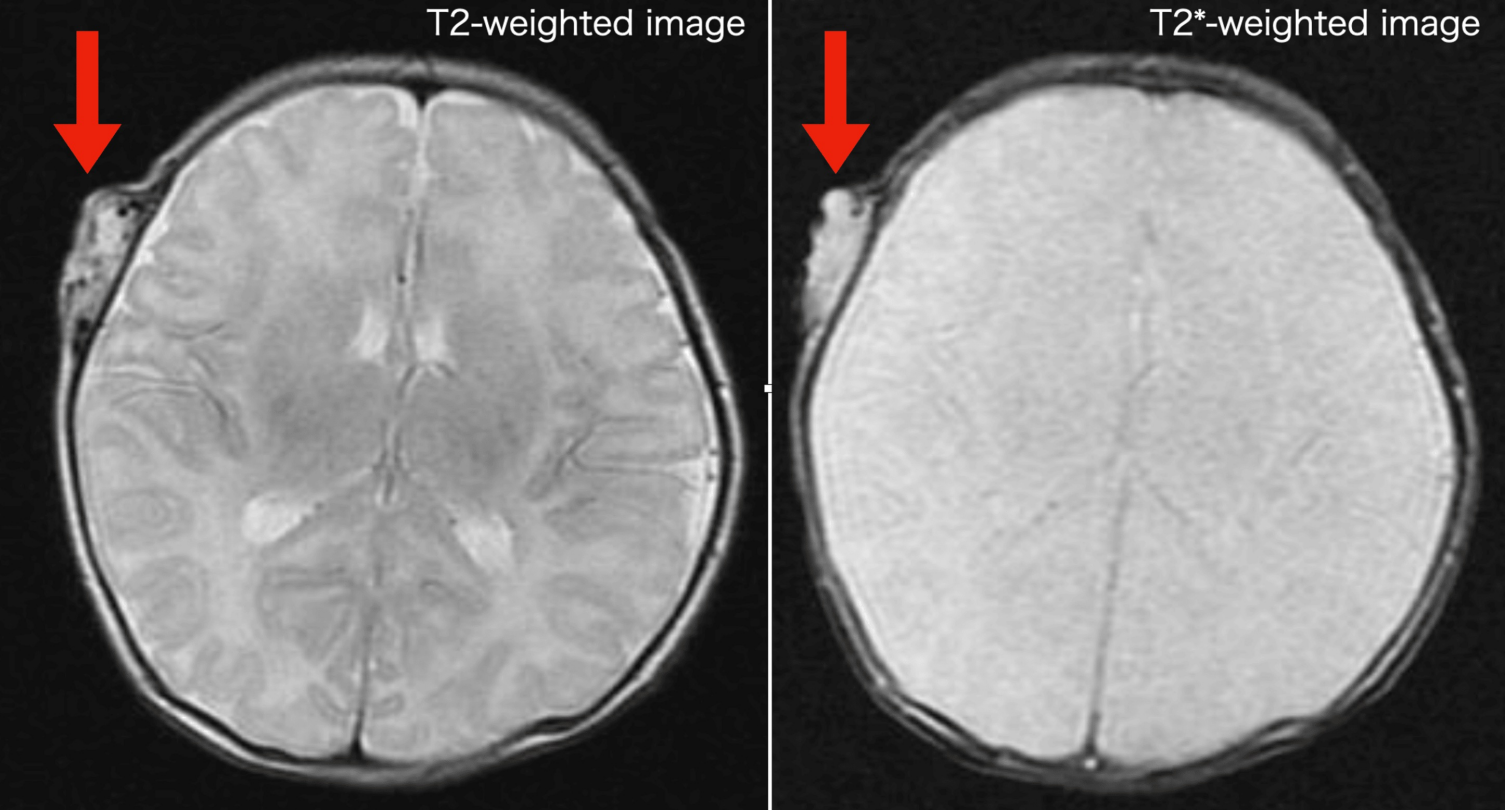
Magnetic resonance imaging with T2-weighted image on the left showed a flow cavity within the mass, with no intracranial or vascular communication. Magnetic resonance imageing with T2*-weighted image on the right showed no flow cavities inside the mass.
